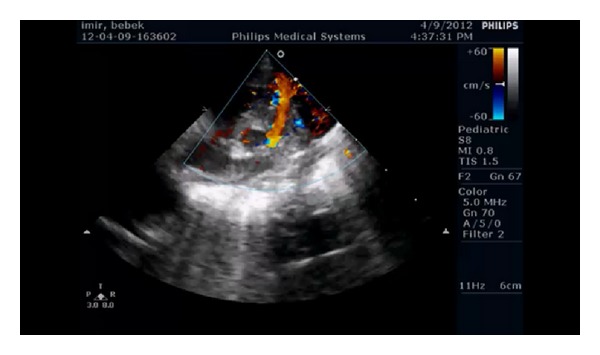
Postnatal echocardiographic examination demonstrating the large arteriovenous fistula connecting the left coronary artery with the apex of the right ventricle.
